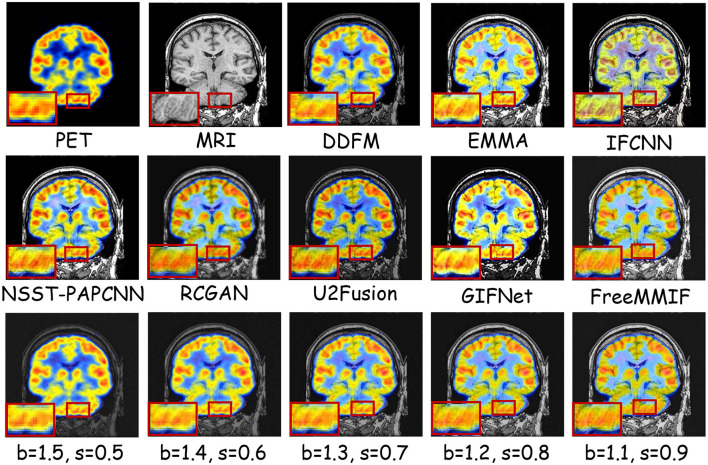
Visual comparison of PET-MRI fusion results.
